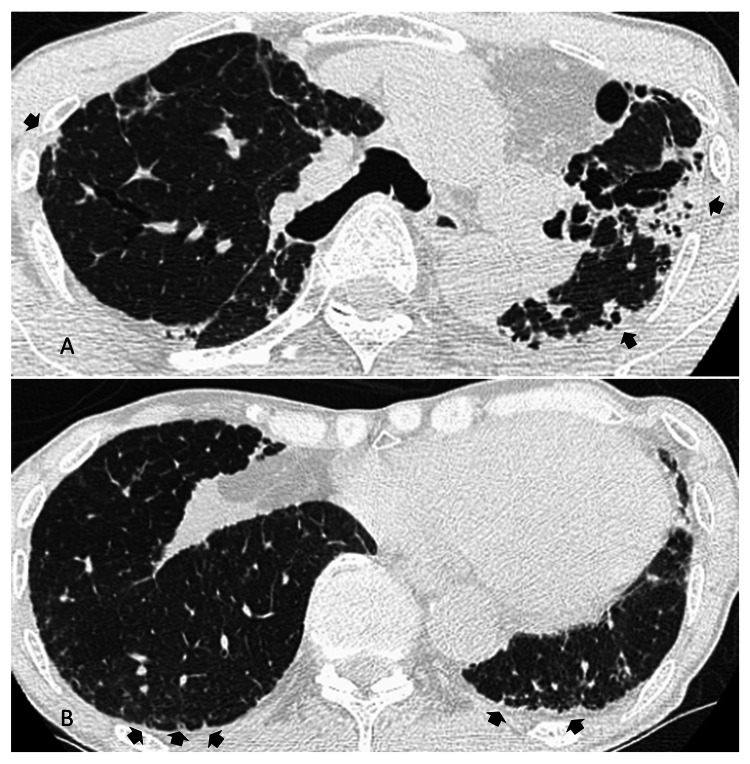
Chest computed tomography images. (A) In the upper-lung zone, parenchymal fibrotic opacities with traction bronchiectasis (arrows) were shown. (B) In the lower-lung zone, lesions are minimal, with only mild reticular changes (arrows) observed at the base of both the lungs.
